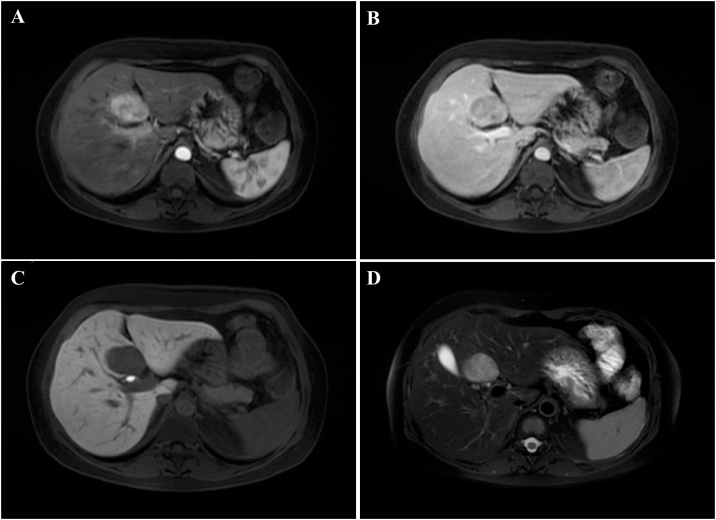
Preoperative MRI scans: T1-weighted image in the arterial phase (A); T1-weighted image in the portal venous phase (B); T1-weighted image in the hepatobiliary phase (C); pre-contrast T2-weighted image with fat-sat.
